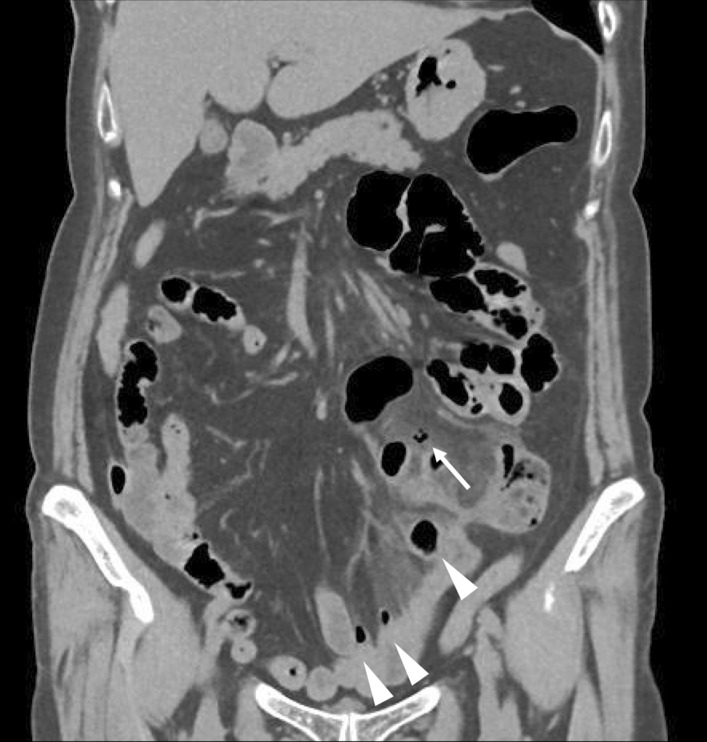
Selected CT images showing multiple diverticula in the small intestine (arrowheads), mesentery inflammatory changes, and extraintestinal air (white arrow)
